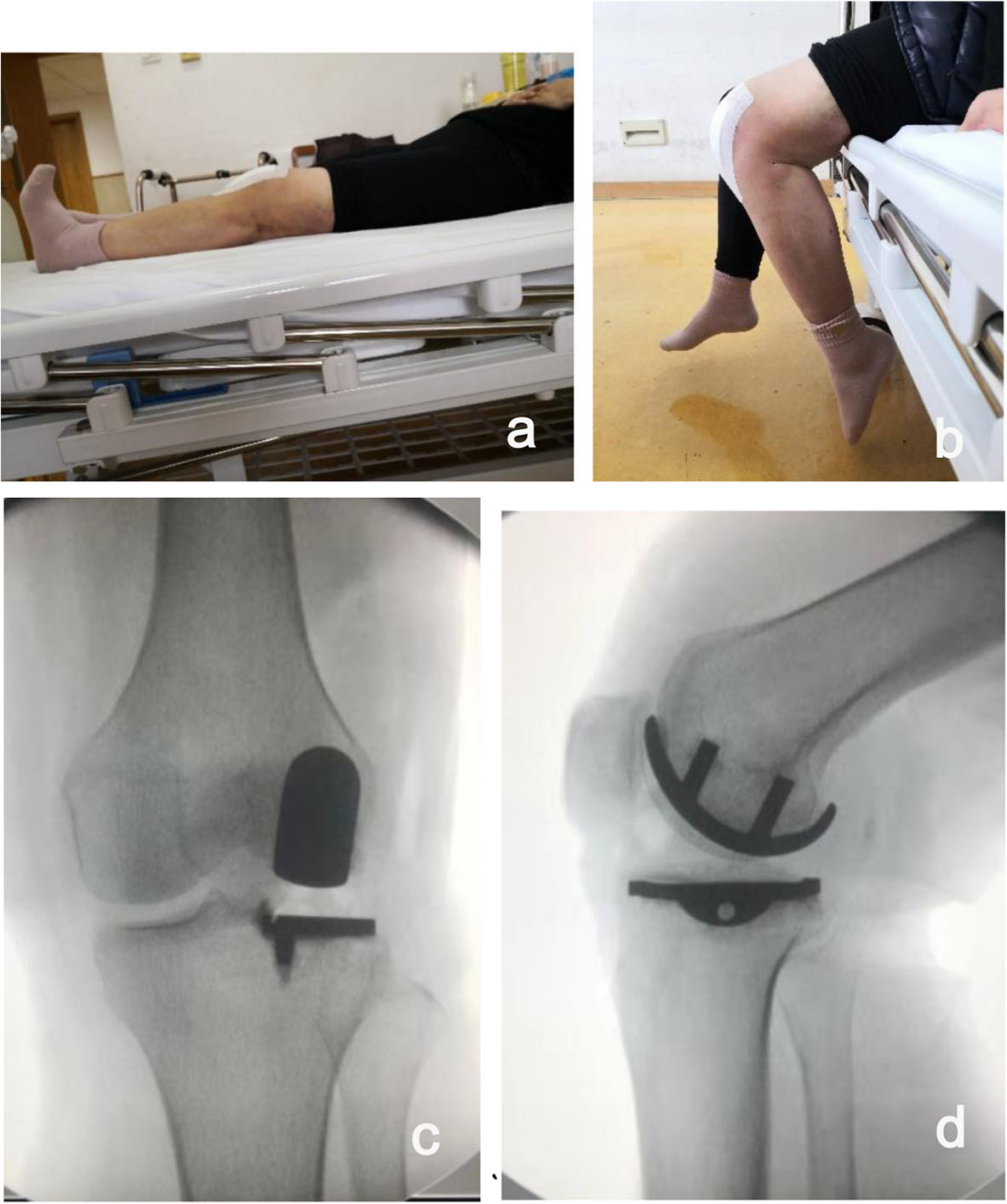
(a, b): Postoperative clinical functions: range of motion was 0° to 90°. (c, d) Postoperative anteroposterior and lateral radiographs showing an optimal size and precise position of the prosthesis
